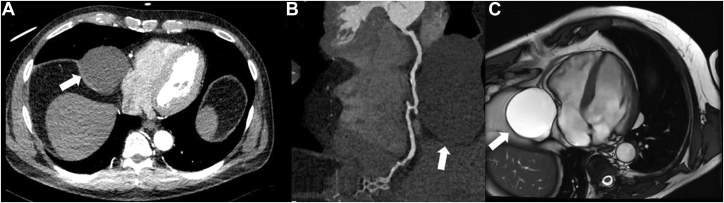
Coronary Computed Tomography and Magnetic Resonance Imaging Coronary computed tomography showed the presence of a large cyst (arrow) adjacent to the (A) right atrioventricular groove and the (B) right coronary artery. There was mild atherosclerosis of the right coronary artery. (C) Cardiac magnetic resonance imaging confirmed mild extrinsic compression of the right atrioventricular groove by the cyst (arrow).
